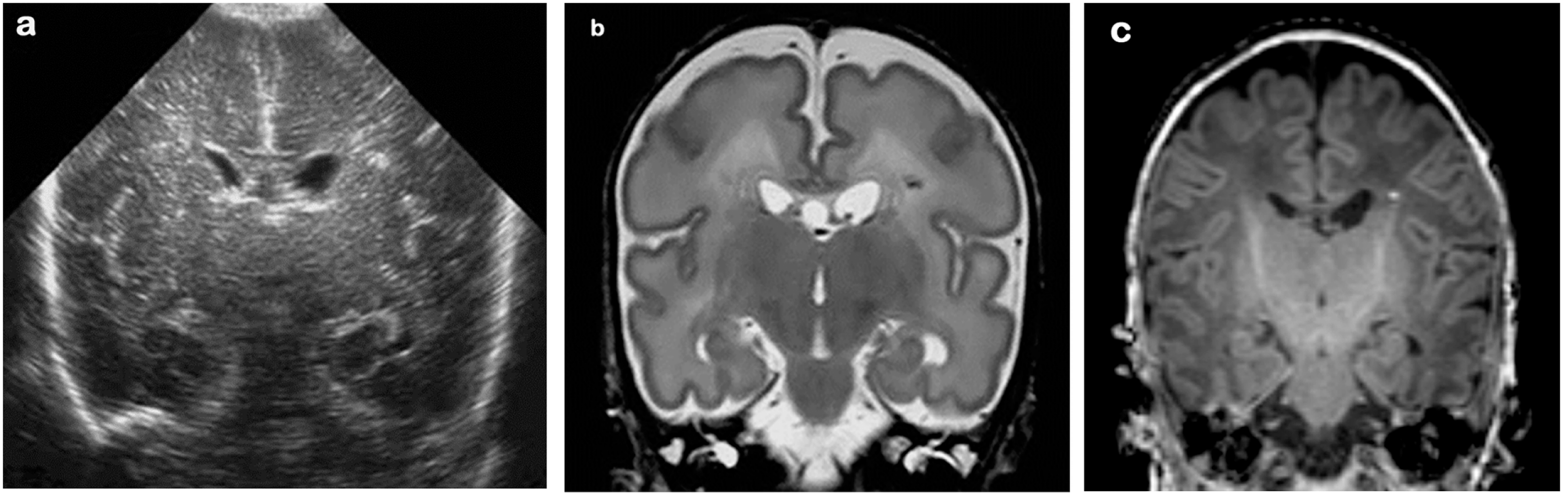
26 week gestation infant; cUS and MRI at 32 weeks post-menstrual age. (a) cUS mid-coronal view, showing a focal lesion in the white matter also seen on early MRI-T2 weighted (b) and at TEA-T1 weighted MRI (c)
